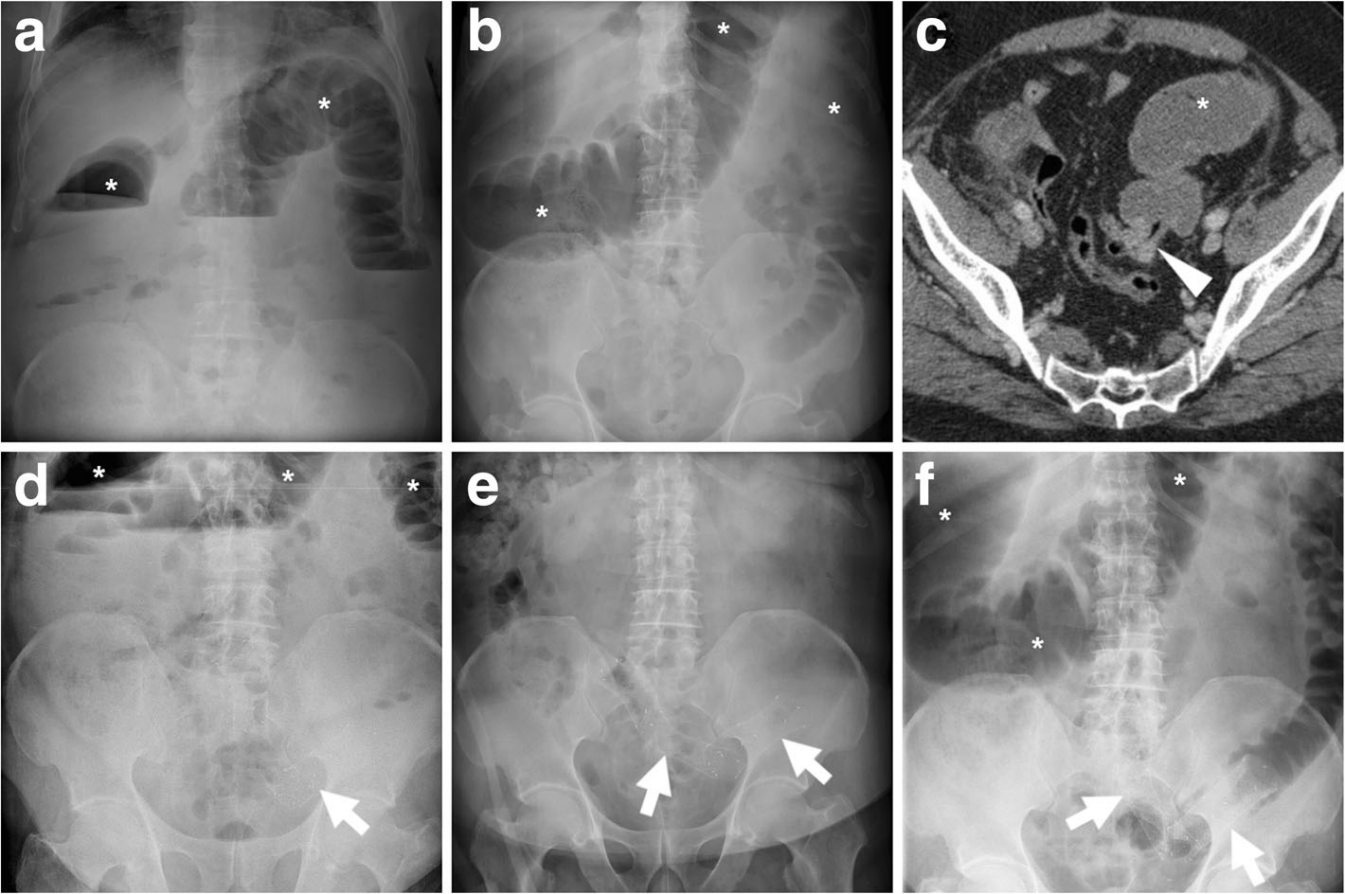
Stent malpositioning causing failed relief of LBO. Initial upright (a) and supine (b) plain radiographs showed dilated colon (*) with air-fluid levels (a) consistent with distal mechanical obstruction. CT (c) and endoscopy confirmed a short “apple-core” stricture consistent with sigmoid CRC (arrowhead in c). A week after the procedure, radiographic follow-up (d-f) showed persistently dilated bowel above two angulated SEMS (thick arrows). Immediate surgery was required to remove pT3N0 CRC
